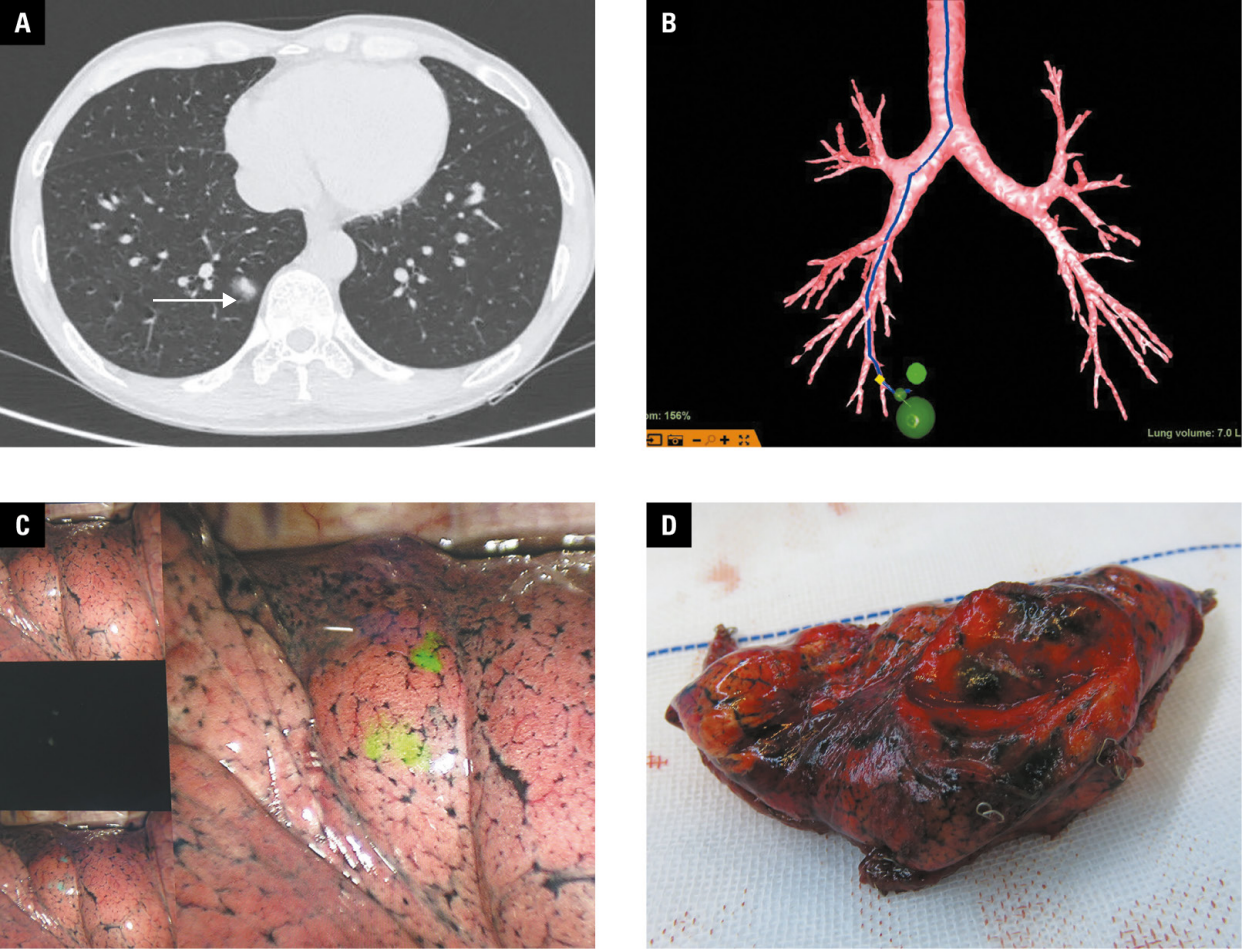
Pulmonary nodule localization using virtual bronchoscopic navigation (VBN); A – computed tomography image showing a small pulmonary nodule (arrow) located in the right lower lobe; B – preoperative design of the VBN localization path with the LungPro software; C – injection of a indocyanine green marker into the corresponding site based on the virtual navigation pathway; D – verification of localization accuracy after thoracoscopic surgery
